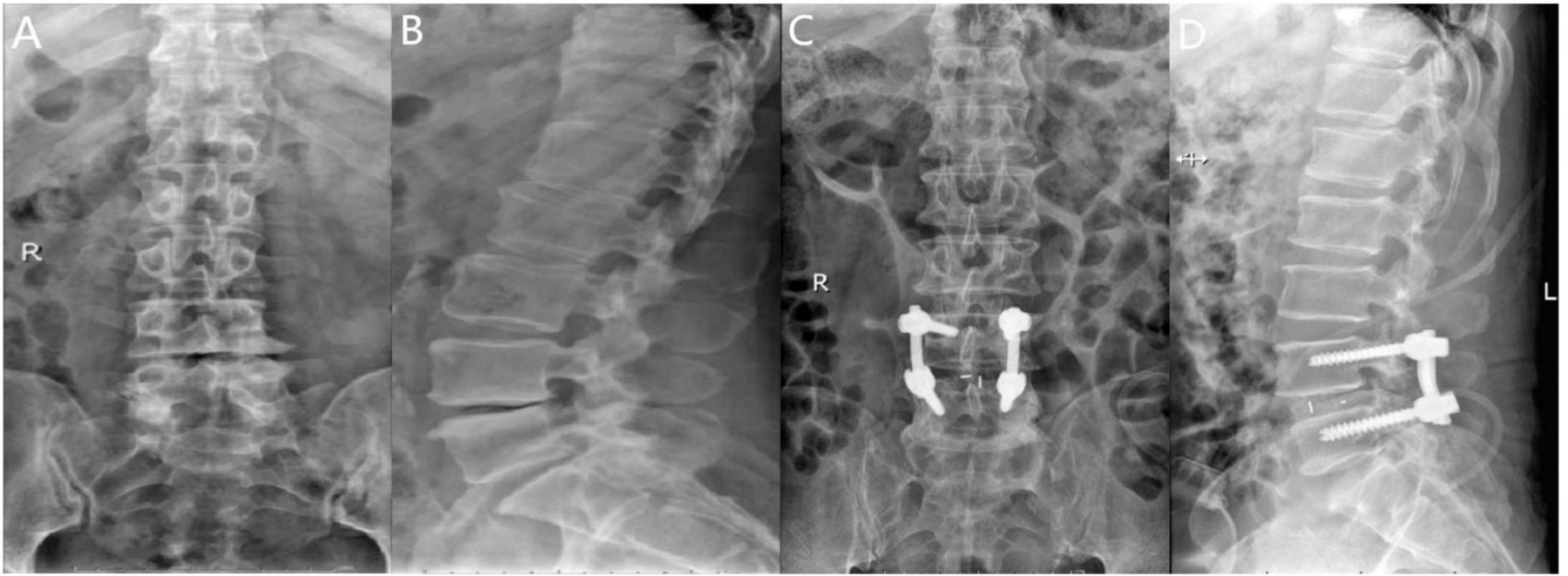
(A) Preoperative anteroposterior radiograph of the lumbar spine; (B) preoperative lateral radiograph of the lumbar spine; (C) postoperative lateral radiograph showing spinal instrumentation; (D) postoperative anteroposterior radiograph showing spinal instrumentation.
